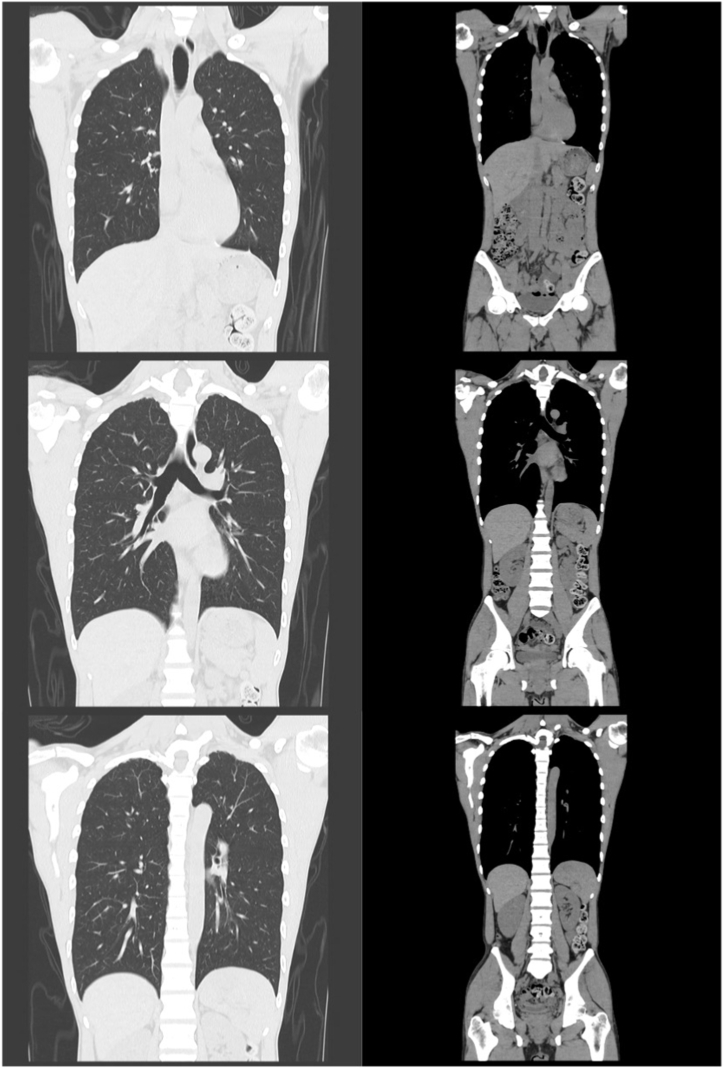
CT before treatment Pre-treatment images. Thoracoabdominal CT scan showed no abnormal findings. There were reports of malignant neoplasms within 3 years of GFAP-A onset, but none were identified in this patient at this time [1,6]. CT, computed tomography GFAP-A, glial fibrillary acidic protein astrocytopathy.
